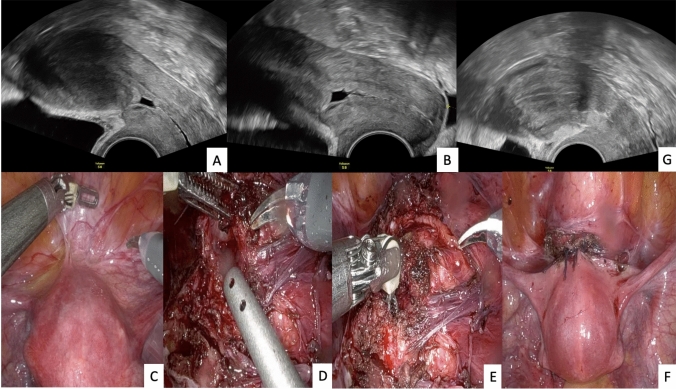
A+B Preoperative transvaginal ultrasound demonstrating an isthmocele. C: Intraoperative view prior to surgical dissection. D: Intraoperative view of the opened isthmocele following excision of the fibrotic niche tissue. E: Placement of a forced grasper within the cranial aspect of the uterine cavity to delineate the defect; the caudal wound margin dissected and mobilised from the urinary bladder (vesicouterine space). F: Completed surgical repair with monolayer closure of the myometrial defect. G: Postoperative transvaginal ultrasound demonstrating restoration of the anterior uterine wall with a residual myometrial thickness of 9 mm
